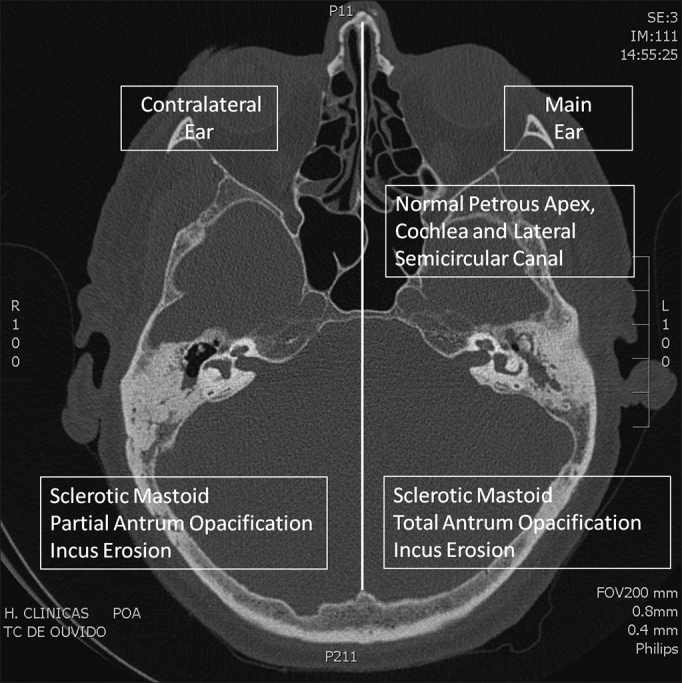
Axial CT Scan. Comparison of abnormalities in the main (left) and contralateral (right) ear.
